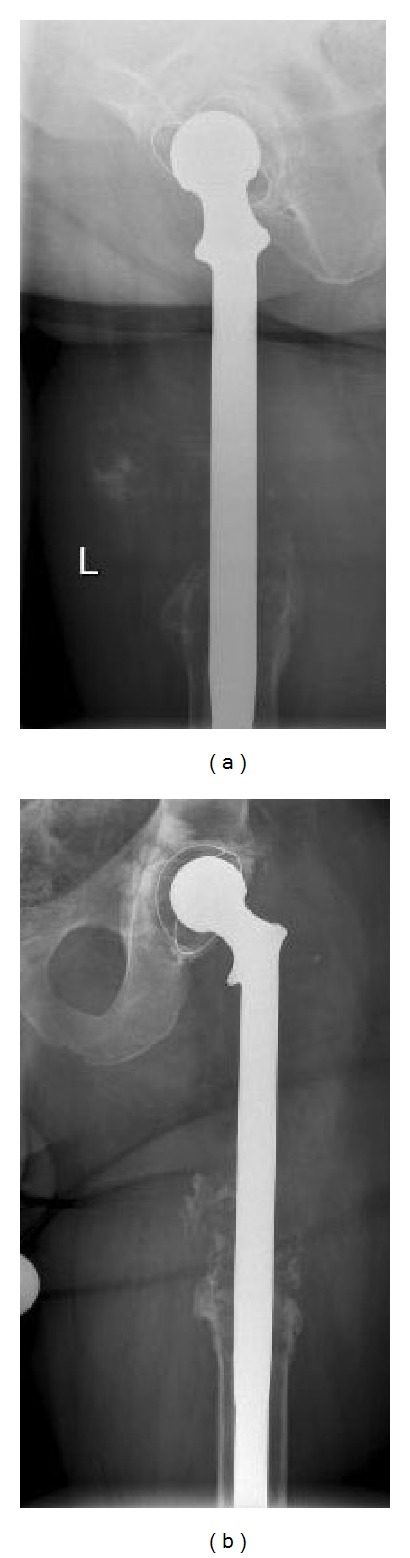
(a) Radiographs showing ectopic ossification on the lateral view revealing local recurrence. (b) AP view.
